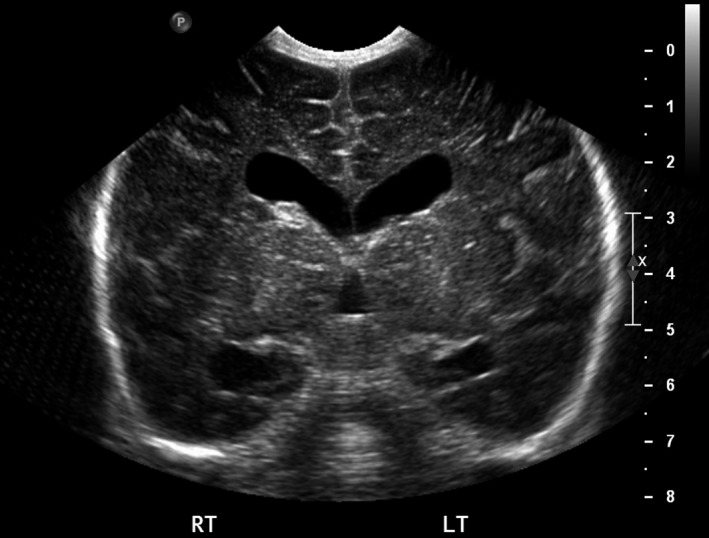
Neurosonogram showed right subependymal hemorrhage and dilation of lateral ventricles.
